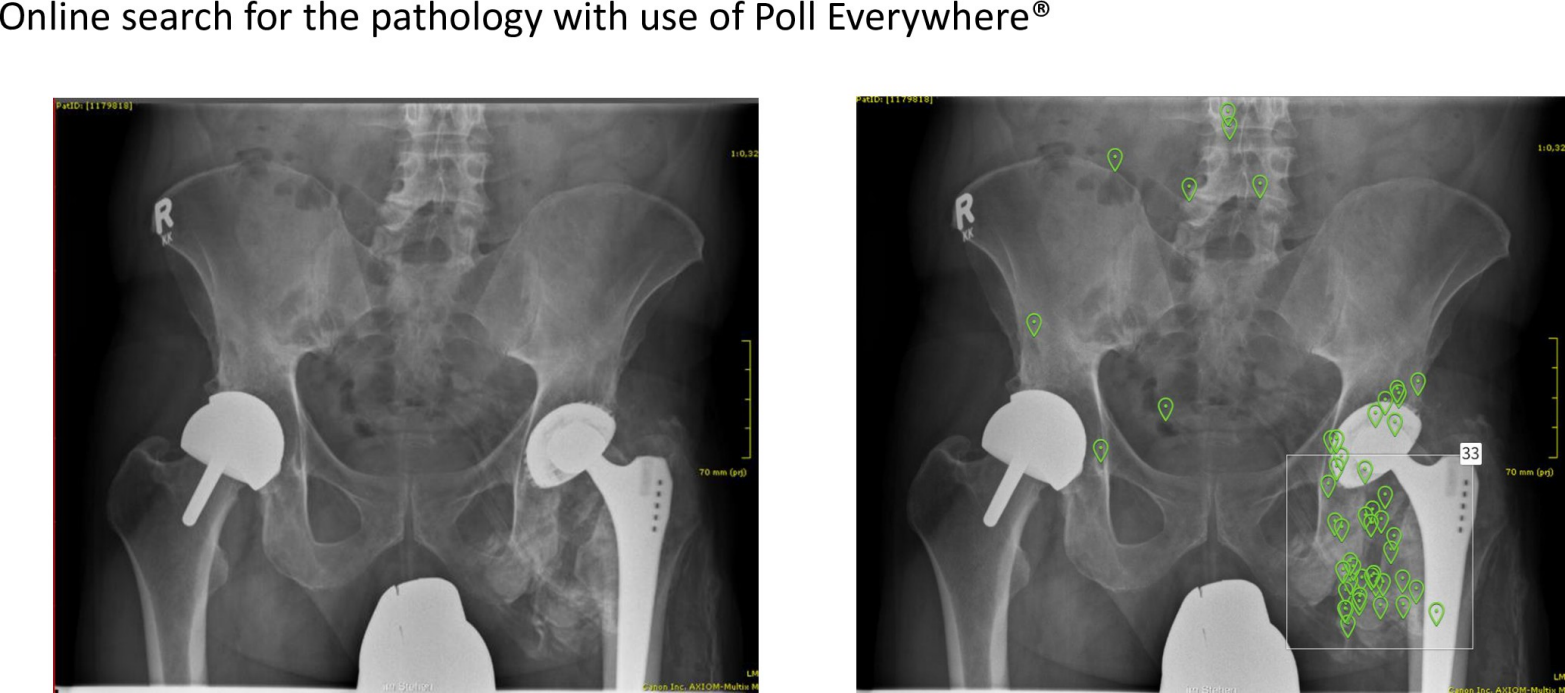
Metallosis in the left hip with typical tissue changes. Live voting with Poll Everywhere®. Green mark corresponds to one student vote. Area of interest is marked with a rectangle. 33 out of 50 (= 66%) students identified the suspected area of metal infiltrated tissue correct (X-ray of the hip by UKGM Radiology Marburg).
